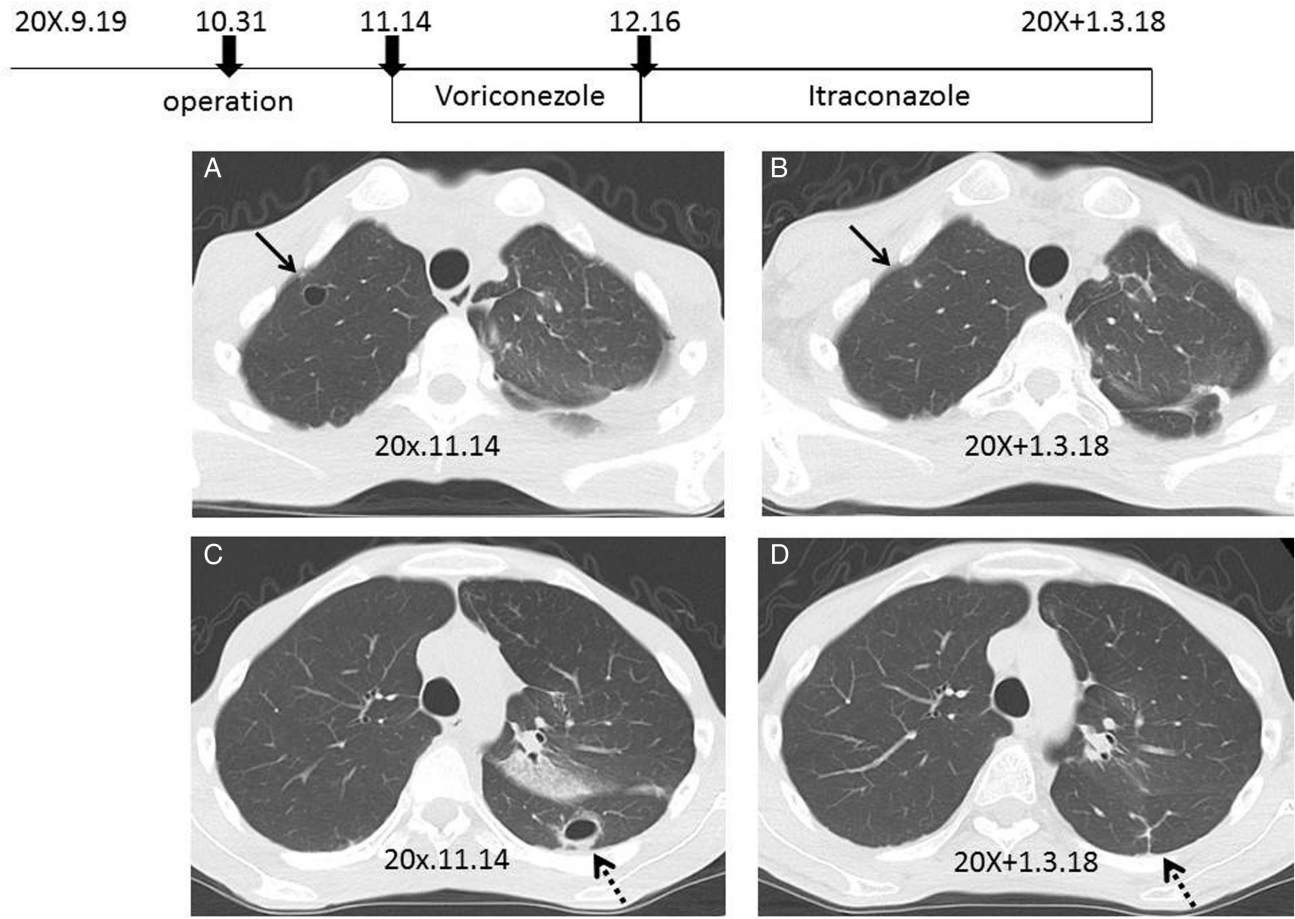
Upper panel shows the clinical course of treatment. (A) Chest computed tomography shows a cyst in the right upper field after partial resection of the left upper lesion. (B) A cyst in right upper field disappeared after antifungal treatment. (C) A cavity in left lower field is seen. (D) The cavity in left lower field became scars after antifungal treatment.
